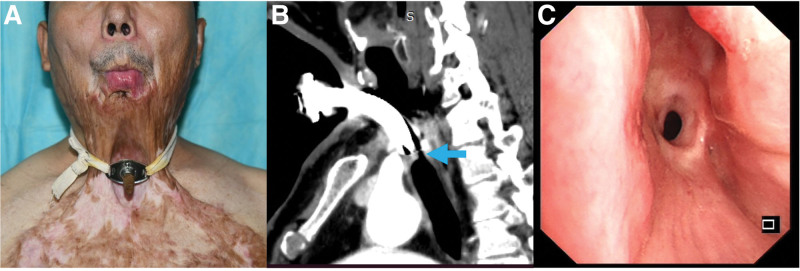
Clinical and diagnostic features of severe postburn neck contracture and complex tracheal stenosis. (A) Microstomia (small mouth) and fixed cervical flexion deformity resulting from severe postburn neck contracture. (B) Sagittal chest computed tomography image demonstrating severe tracheal stenosis (blue arrow) below the tracheostomy stoma site, with the narrowest diameter measuring 6 mm. (C) Bronchoscopy view confirming complex post-tracheostomy tracheal stenosis (bottleneck sign) below the stoma site.
